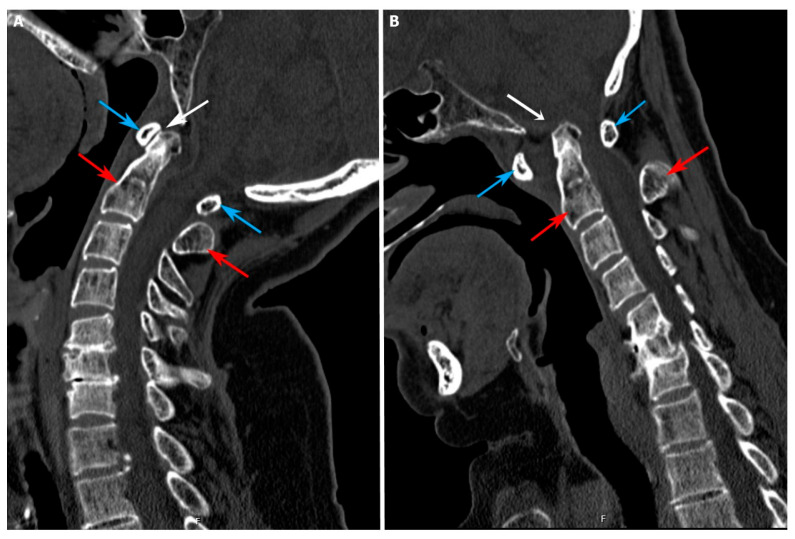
Atlantoaxial dislocation on dynamic computed tomography (CT) imaging. (A): Extension view; (B): flexion view. White arrow: change in the atlantodental interval (ADI); Blue arrow: C1 vertebra; Red arrow: C2 vertebra.
